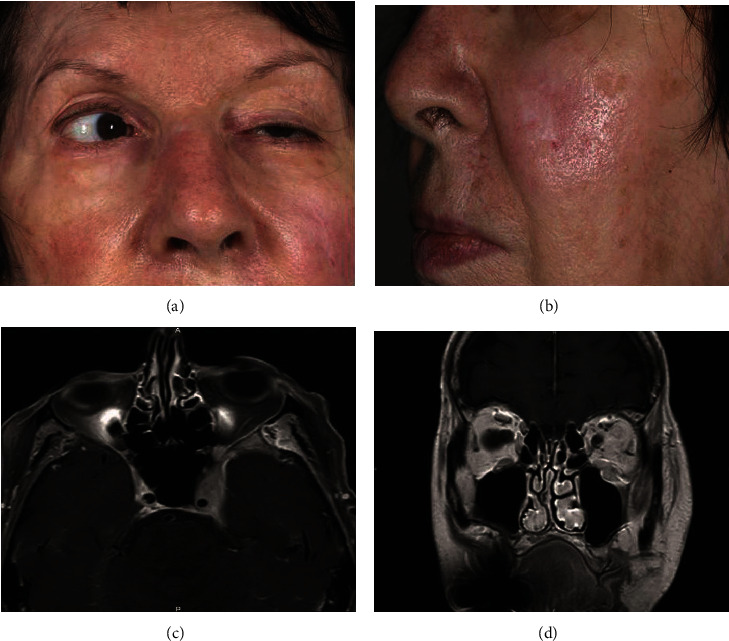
(a) Complete left ophthalmoplegia and left upper-eyelid ptosis. (b) Actinic keratosis of the left cheek. (c) Facial and orbital MRI: sagittal view: infiltrating lesion extending through the left infraorbital canal orbital apex and the left cavernous sinus with perineural infiltration. (d) Facial and orbital MRI: coronal view: infiltrating left orbital process started from the left cheek with a large amount of necrosis.
